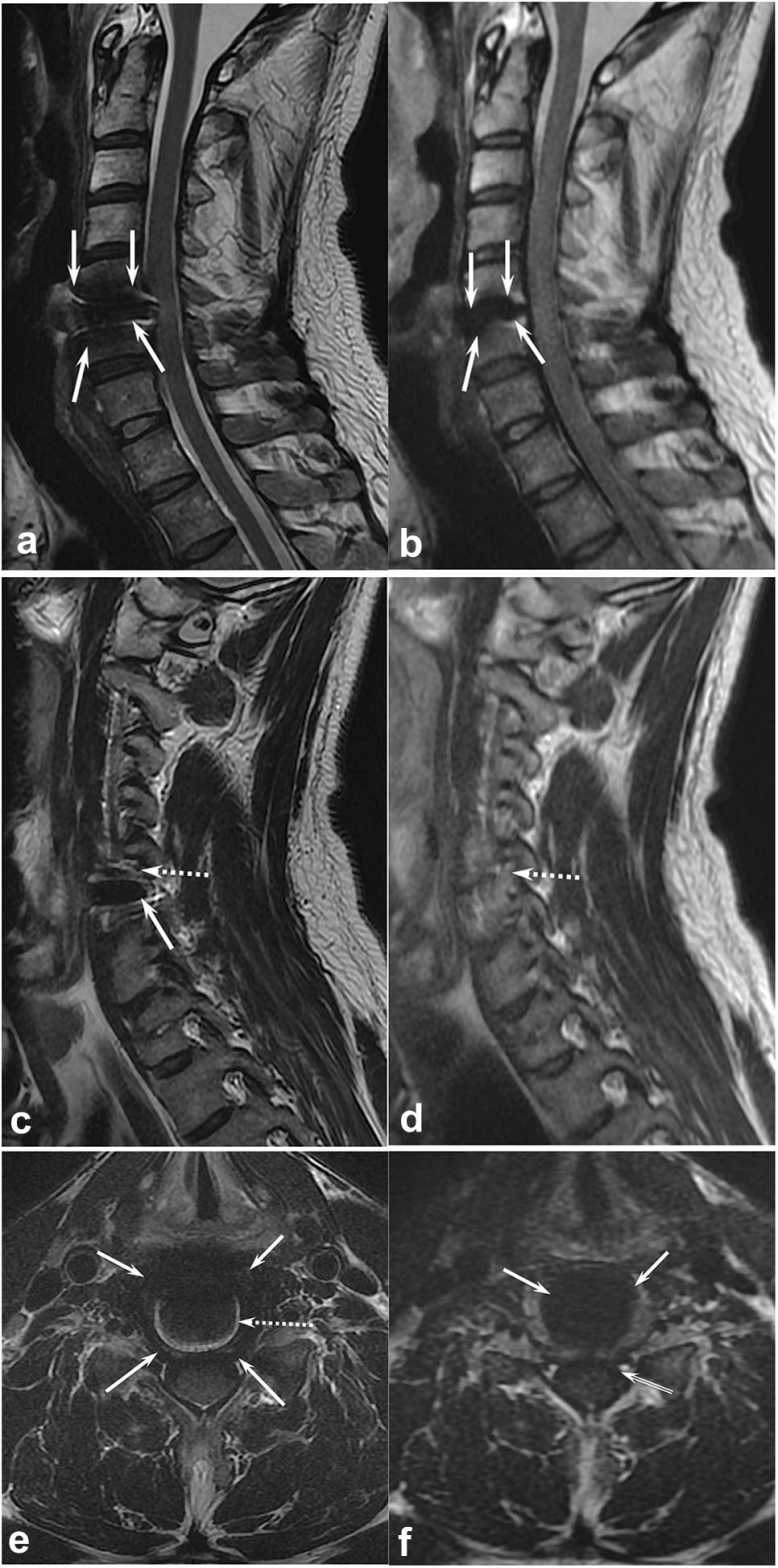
Comparisons of T2-weighted sequence (T2WI) (a, c, e) and multiacquisition with variable resonance image combination selective T2-weighted sequence (MAVRIC SL T2) (b, d, f) of the cervical spine of a volunteer (V3). (a) Mid-sagittal T2WI shows a metallic artifact (arrows) that obliterated anterior paravertebral soft tissue, upper and lower vertebral bodies, and anterior surface of the spinal cord. (b) Mid-sagittal MAVRIC SL T2 showed a markedly decreased signal void area (arrows) with nearly no geometric distortion and minimal signal pile-up. However, severe blurring was observed in the spinal cord. (c) Parasagittal T2WI at the left foramen level shows a signal void (arrow) obliterating more than half of the neutral foramen. Note the mild geometric distortion of the nerve root (dashed arrow). (d) Parasagittal MAVRIC SL T2 at the left foramen level visualized completely normal neutral foramen with no metallic artifact and no distortion of the nerve root (dashed arrow). (e) Axial T2WI at the operated level shows signal voids (arrows) obliterating the anterior subarachnoid space, anterior portion of the spinal cord, anterior paravertebral soft tissue, and both neutral foramina. Note the signal pile-up artifacts (dashed arrow). (f) Axial MAVRIC SL T2 showed a marked decrease in signal void (arrows) and almost no signal pile-up artifacts. However, the anterior subarachnoid space is obliterated by the low signal intensity of the cerebrospinal fluid pulsation artifact (double lined arrow). Severe blurring was noted in the spinal cord, with decreased T2 contrast.
